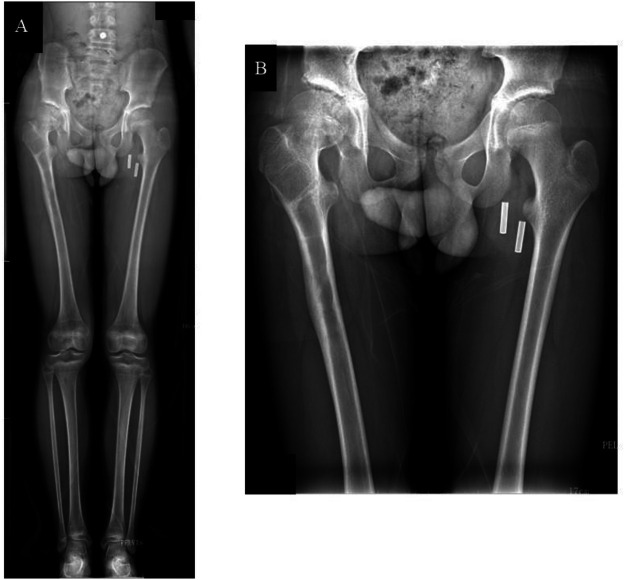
At the 1.5-year postoperative follow-up, full-length radiograph of both lower extremities (A,B) showed maintained equal leg length, well-remodeled union at the femoral fracture site, and preserved normal femoral head morphology with no signs of AVN.
